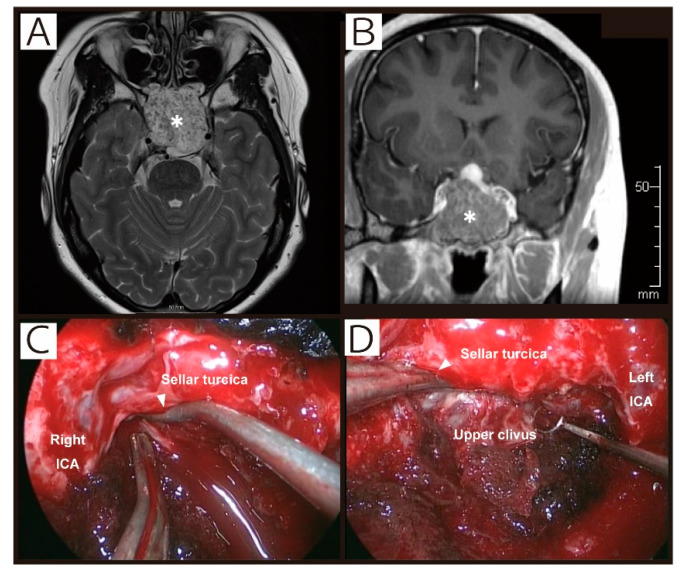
Patient #3. A 38-year-old woman presented with left visual impairment and abducens nerve palsy. MRI ((A), T2-weighted image; (B), contrast-enhanced T1-weighted image) demonstrated a large skull base mass extending to the clivus, sphenoid sinus, bilateral petrous apex and cavernous sinus. The pituitary gland was compressed upward, but the interface between the dura and tumor was preserved. During the extended transsphenoidal approach, tumors at the posterior cavernous sinus ((C), intraoperative image) and dorsum sellae ((D), intraoperative image) were exposed and removed with retraction of the dura of the pituitary gland upward by suction tube. White arrowheads show the point of extradural retraction. The pathological diagnosis was chordoma. The postoperative course was uncomplicated, but the patient developed nausea and severe fatigue, and turned out to have severe hyponatremia (serum sodium level, 126 mmol/L) on POD 7 with a weight gain pattern (2.4% increase from baseline). Sodium level improved to 132 mmol/L on POD 8 only with oral salt supplementation, and symptoms resolved accordingly. *, Tumor.
